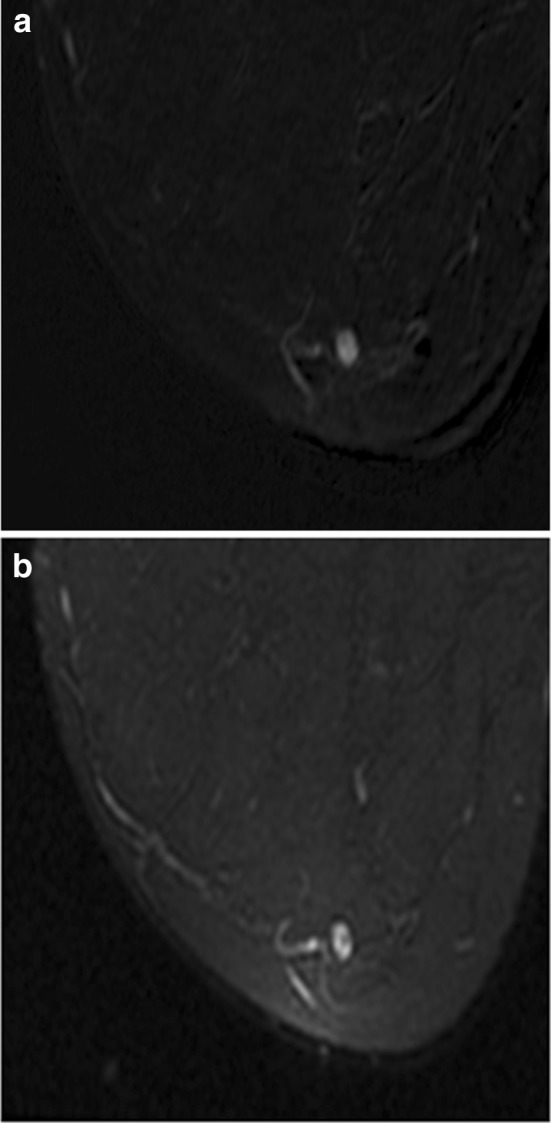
Mass with oval shape and circumscribed margins. a Postcontrast subtraction T1-weighted image shows a 0.6-cm oval-shaped mass with circumscribed margins and homogeneous internal enhancement, which demonstrated high signal on T2-weighted sequence (b) and a BI-RAD 3 assessment was given. 6-month follow-up MRI showed that this mass was stable and is likely an intramammary lymph node. This example shows that BI-RADS 3 assessment is appropriate for masses with an oval shape and circumscribed margins on baseline examination
